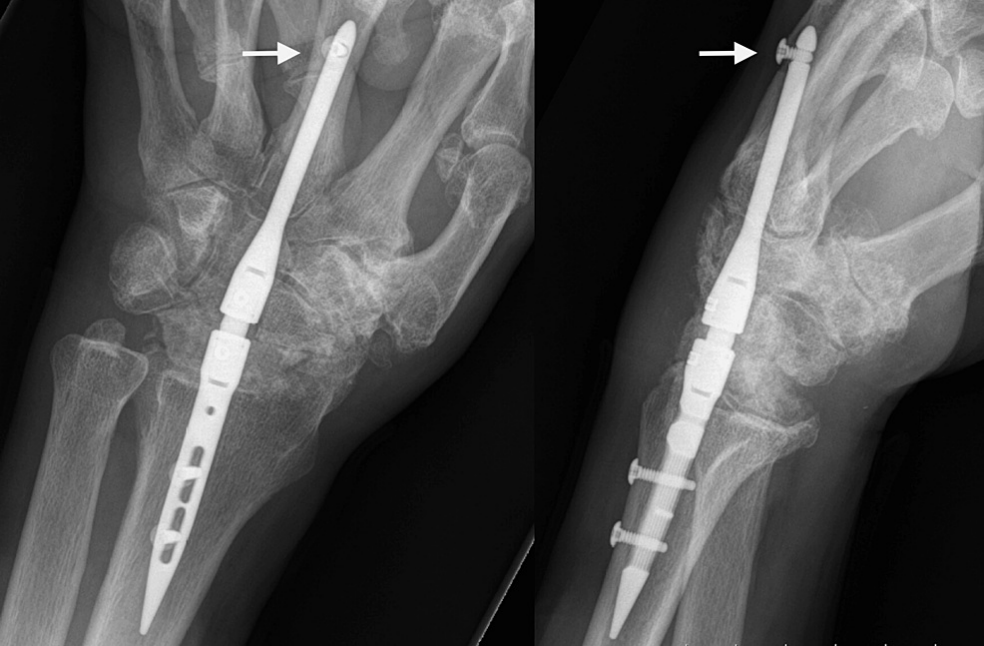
Immediate postoperative radiographs of Case 3 Posteroanterior and lateral views after total wrist arthrodesis using IMPLATE intramedullary nail and proximal row carpectomy. The white arrows show the proper positioning of the distal screw.
